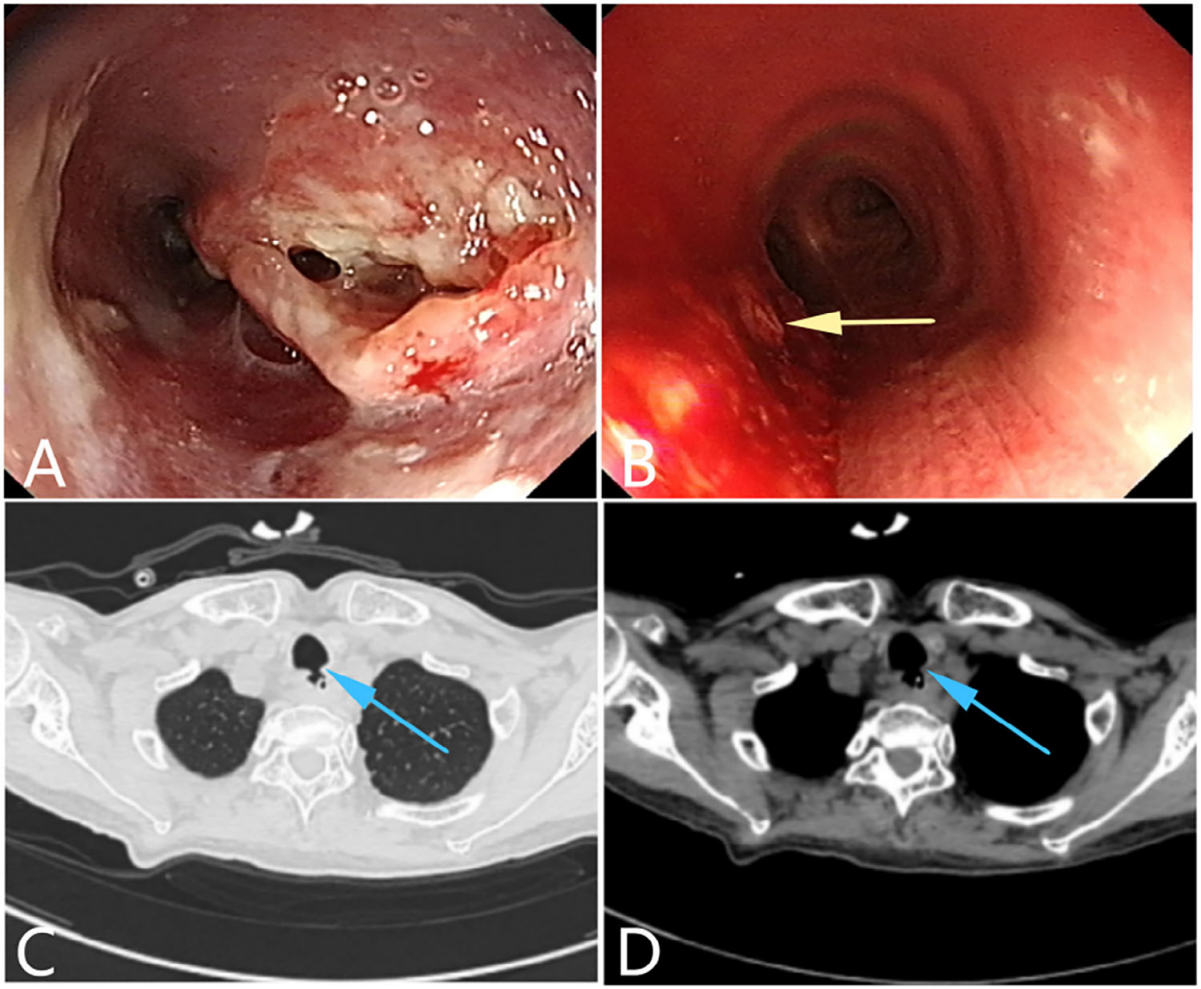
An esophageal malignant tumor invades the trachea, leading to a tracheoesophageal fistula. A) Gastroscopy revealed an ulcerative malignant tumor in the esophageal cavity. B) Bronchoscopy showing the formation of a fistula on the left wall of the trachea with necrotic material and blood flowing out from it. C, D) CT scan showing the pathway between the esophagus and the trachea. (Original work created by the authors).
